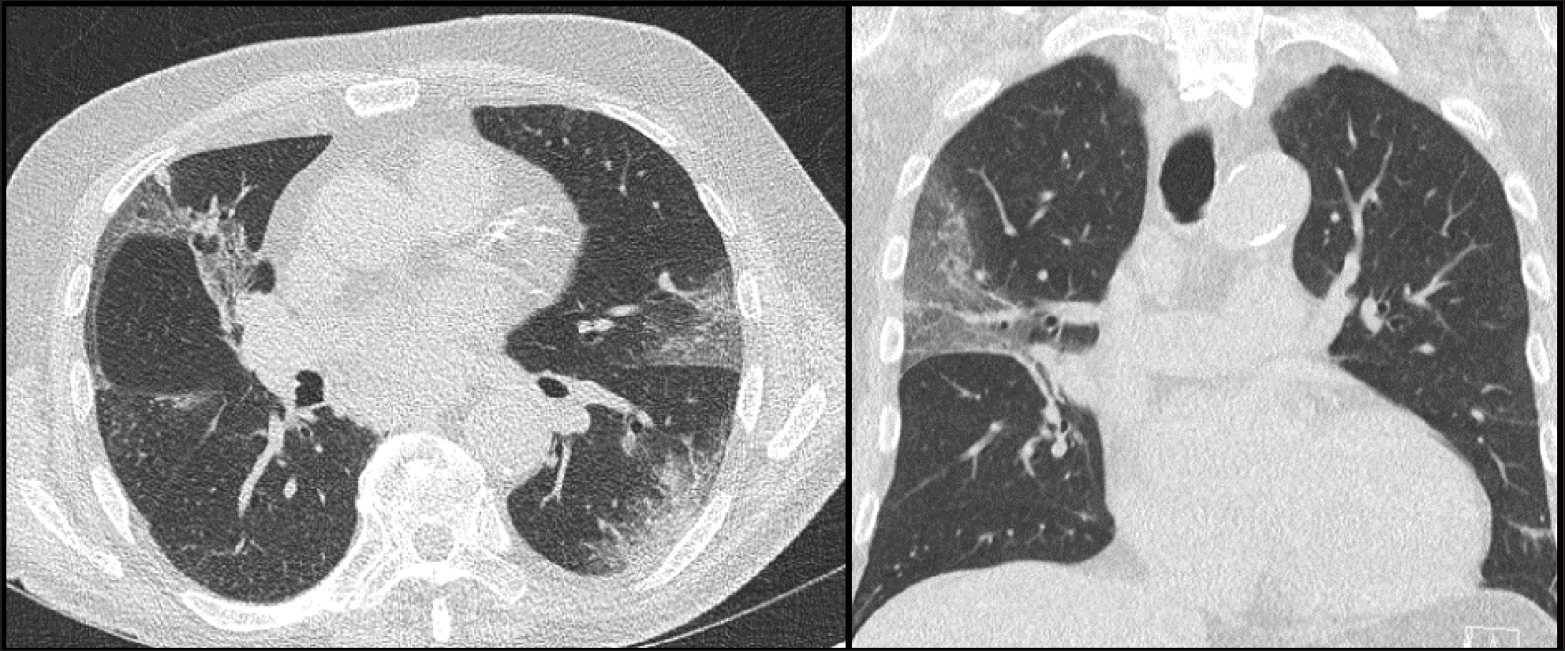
Low-dose chest CT with findings suggestive for COVID-19 pneumonia, showing multifocal, bilateral peripheral ground-glass opacities and consolidations.
